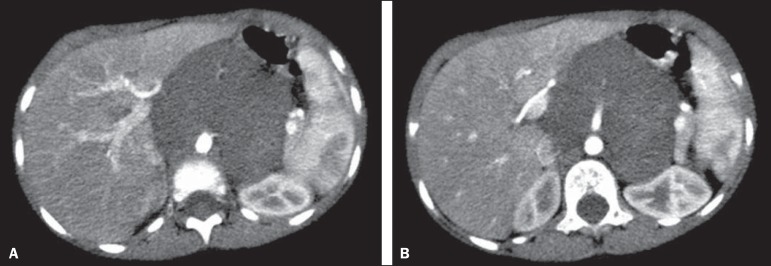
A: Axial CT scan with intravenous and oral contrast of a patient with a
